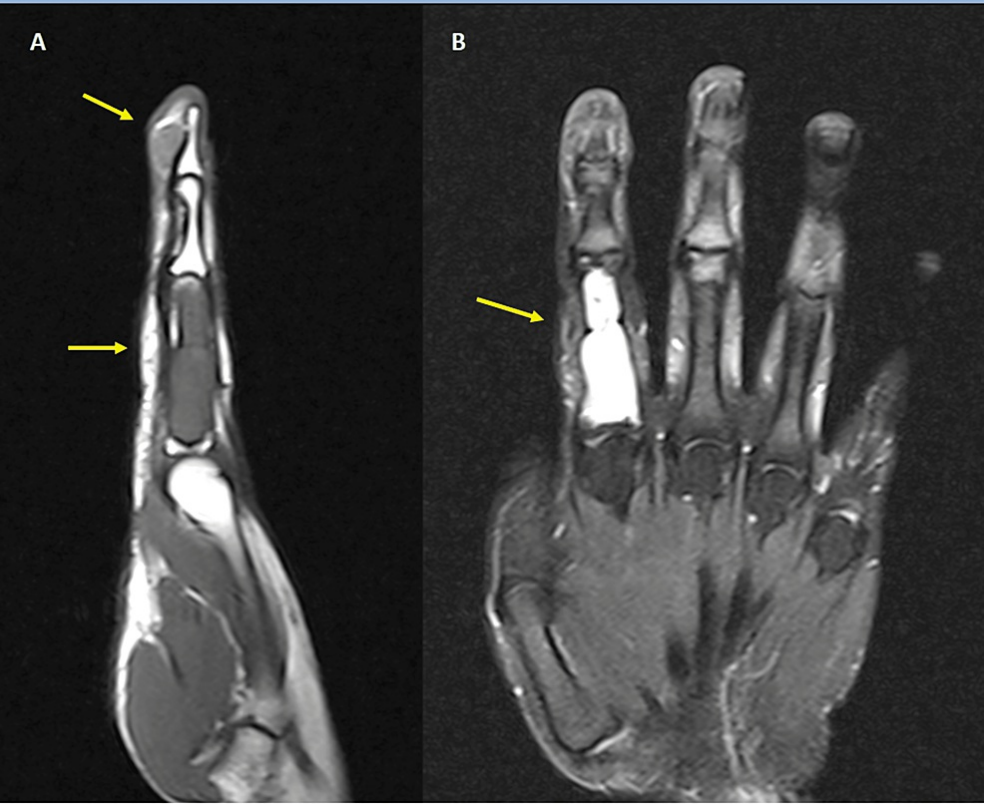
T1-weighted (A) and T2-weighted (B) MRI images of the index finger
